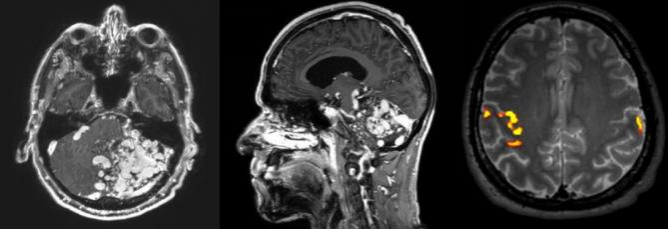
Cerebral MRI with gadolinium: A large AVM is centered in the left cerebellar hemisphere extending towards the middle cerebellar peduncle and left lateral margin of the pons. It has afferent branches of the basilar trunk, right vertebral artery and left posterior cerebral artery, and efferent venous branches projected towards the vein of Galen and left transverse sinus. It generates compression and displacement of the brainstem to the right and compression of the left trigeminal nerve and the left statoacoustic bundle. On the right, functional MRI showing right motor cortex.
